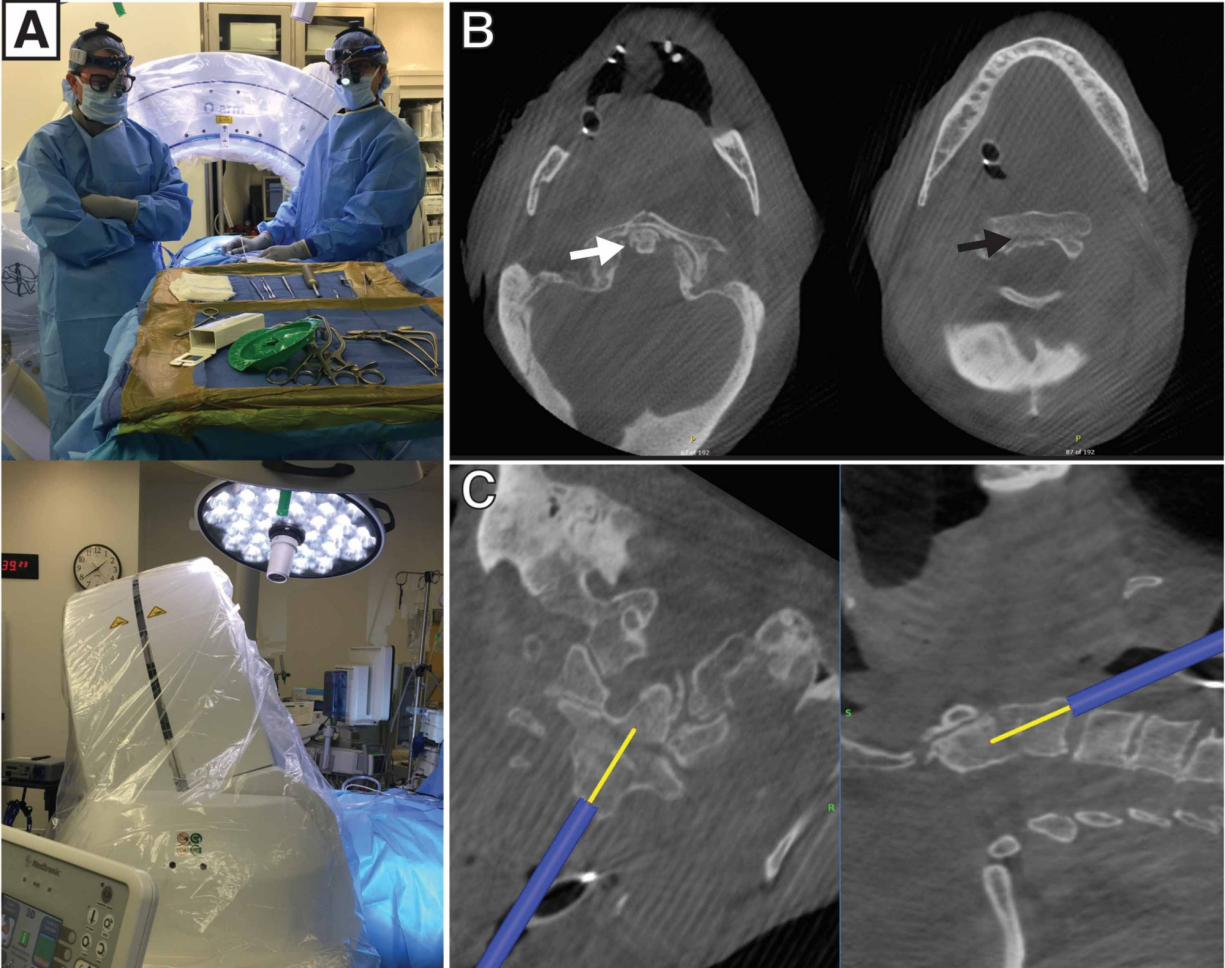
An intraoperative CT provides a real-time view of the fracture and spinal alignment after the patient positioning. The CT is then used to plan and navigate screw placement (A) O-arm set-up. (B) axial images demonstrating intraoperative visualization of the tip of the dens and C2 body, indicated by white and black arrows respectively, prior to screw placement. (C) visualization of screw trajectory CT: computed tomography
